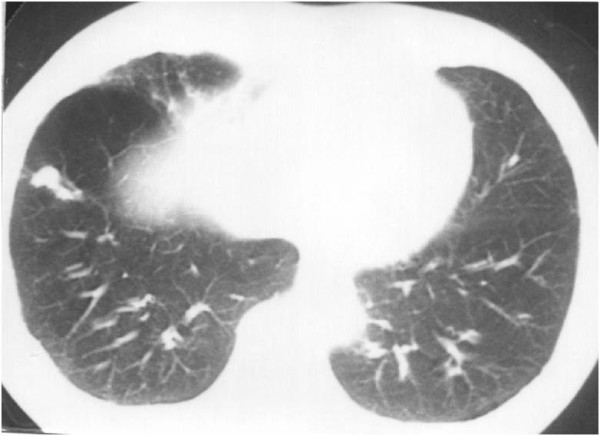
Chest CT scan after radiation therapy. Both lung metastases had shrunk under radiation therapy.
